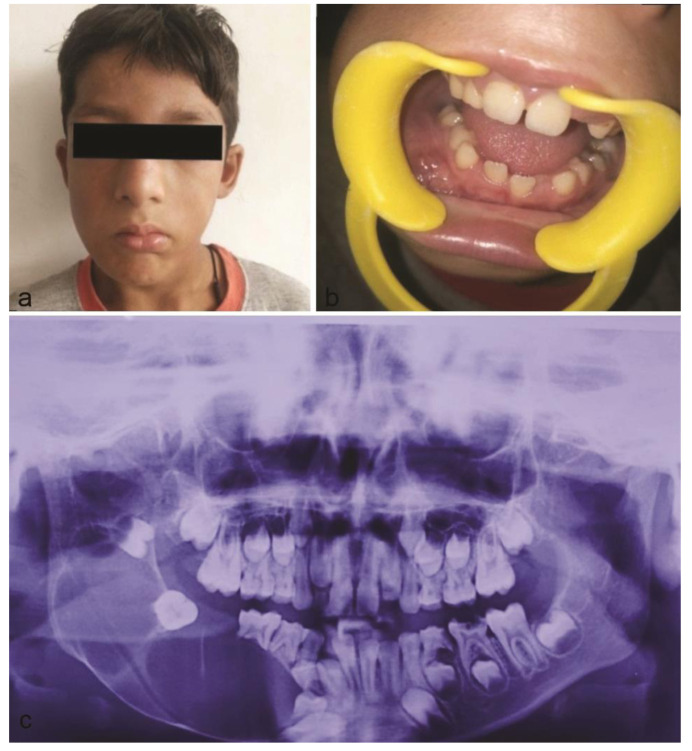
a: Extra oral image of patient showing diffuse swelling on right side of the face, b: Intraorally obliterating swelling present in right buccal vestibule, c: A panoramic radiograph showing well-defined multilocular radiolucency with respect to right mandibular posterior region associated with knife-edge pattern of root resorption in 85 and 46
